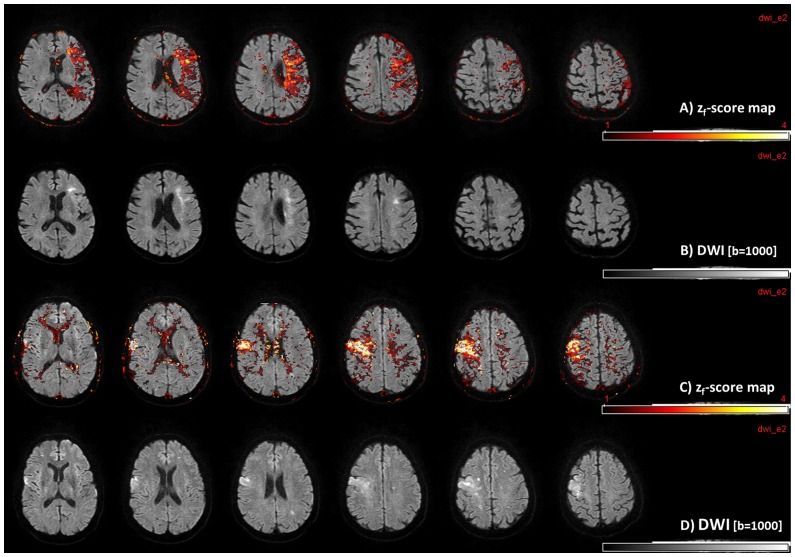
Exemplary use of TDC-modeling. TDC-modeling adapts thresholds automatically, since the same z_f_-score corresponds to individually different absolute thresholds. The TDC interval: [+1< = z_f_< = +4] (overlain with correlated diffusion weighted imaging [DWI]) is shown in rows A and C. Areas colored in yellow in the TDC-maps display z_f_-scores> = +2 (putative critical perfusion), while red colored regions mark areas with z_f_-scores <+2 (putative tolerable hypoperfusion). In rows A and B acute cardioembolic stroke is depicted, where only few yellow areas with critical perfusion (row A) match well with the DWI-alterations (row B). The same behavior was found in the patient shown in rows C and D, who suffered thromboembolic stroke due to acute occlusion of the internal carotid artery on the right and a high grade stenosis on the left side. Again a good match of the TDC prediction (row C) with real ischemic injury in DWI-images (row D) on both sides was found. Note that although the same z_f_-scores were used in both cases and no severe overestimations for critically perfused areas occurred, the automatically calculated individual critical thresholds were 9.7 s for case 1 (rows A & B) and 5.8 s for case 2 (rows C & D).
